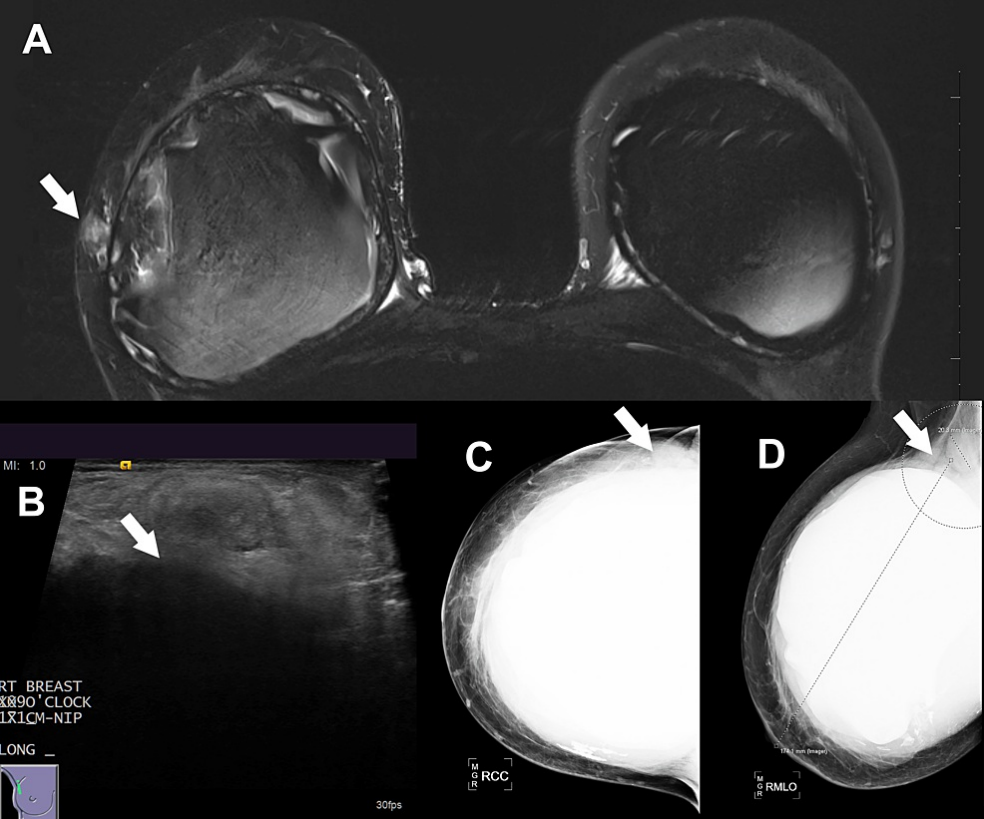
(A) Magnetic resonance imaging with enhancement at the 9 o’clock position of the right breast. (B) Ultrasound with a snowstorm appearance consistent with silicone. (C) Craniocaudal and (D) mediolateral oblique mammogram views showing possible extracapsular silicone and a dominant right axillary lymph node.
